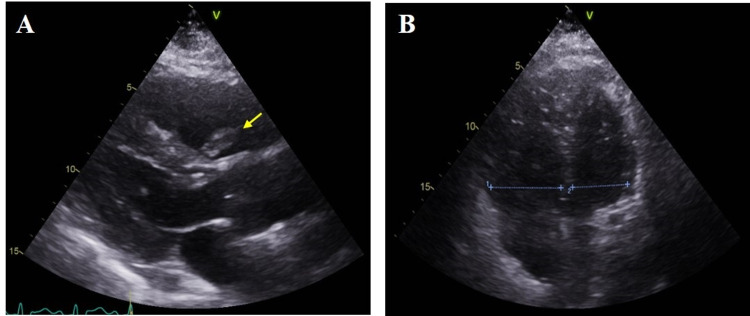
Representative ultrasound images (A) Right ventricular (RV) embolus entangled within the tricuspid apparatus (yellow arrow). Also showing minimal compression of left ventricle (LV) due to elevated RV pressures. (B) Apical four-chamber view showing an enlarged/dilated right ventricle, representing right heart strain.
